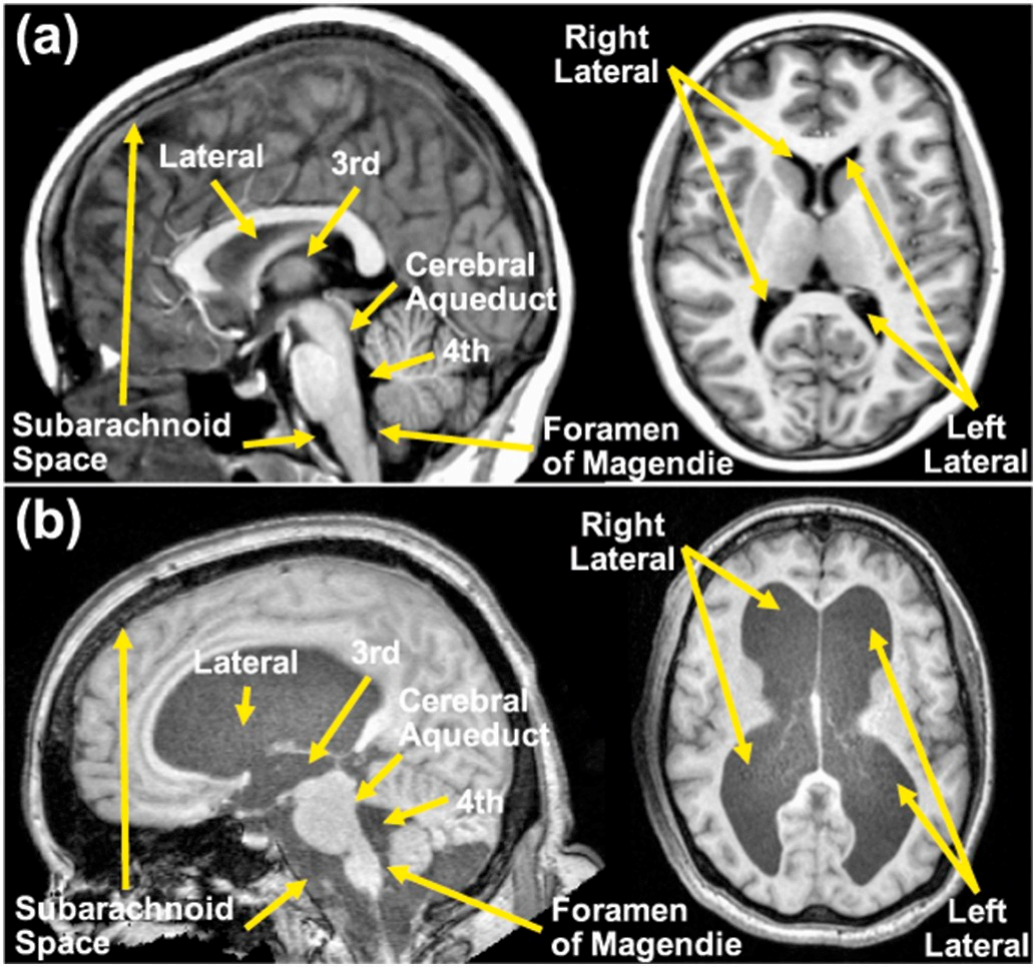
The ventricular system on an MPRAGE T1-weighted MRI of (a): a healthy subject and (b) an NPH subject.
